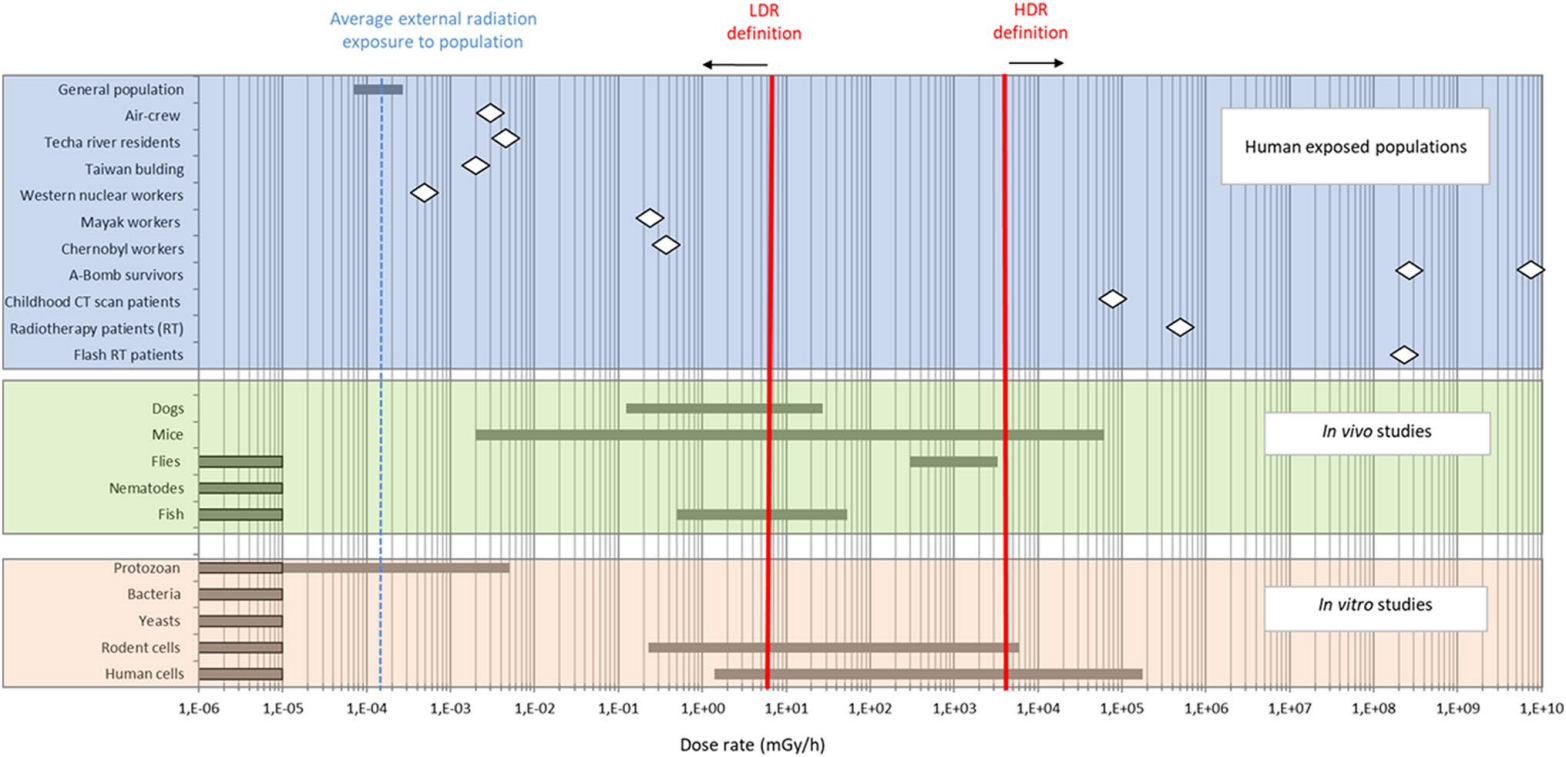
Representation of dose rate ranges (log scale in mGy/h) considered by the different studies presented separately for human, in vivo and in vitro studies. The range of external dose rates received in the general population is shown along with the average 2.4 mGy/h exposure rate worldwide (blue dashed line) (UNSCEAR 2000). LDR definition corresponds to 5 mGy/h and HDR to 0.05 Gy/min (solid red lines). For epidemiological studies, average dose rates in specific situations are shown and represent radiation exposure above background (white diamonds). In comparison, dose rates from the LSS (given in terms of free-in-air kerma) are large and of the order of 1.9 × 10^8^ mGy/h–8.6 × 10^9^ mGy/h, for atomic-bomb survivors located at 2000 m and 1000 m distance from the Hiroshima hypocentre at time of the incident, respectively (for details, see text). For medical exposure situations, average dose rates to the tumours have been considered for radiotherapy and to the area of the body explored for CT-scan. Note that for exposures of atomic-bomb survivors and patients due to diagnostic and therapeutic procedures, times of exposure are short and, therefore, dose rates given in terms of mGy/h may be misleading. For in vivo and in vitro studies, a range is shown that is representative of the dose rates used in selected publications discussed. Data from experiments carried out in Deep Underground Laboratories (DULs) are also reported (grey bars between 1 × 10^–6^ and 1 × 10^–5^ mGy/h)
